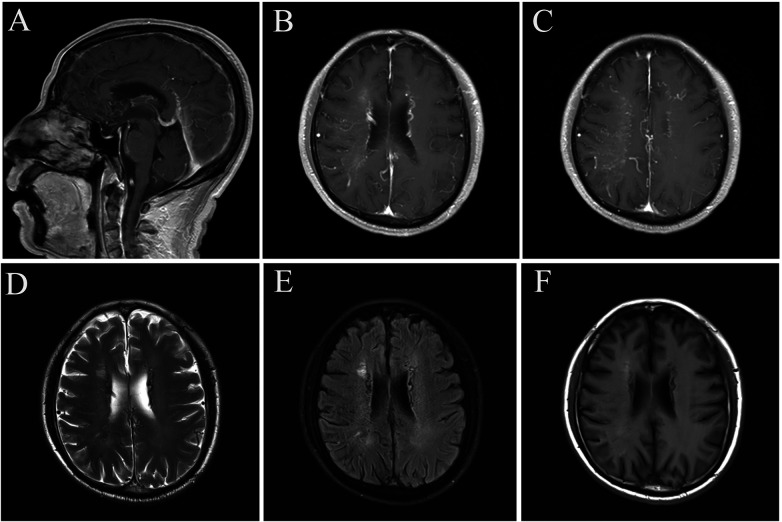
(A–F) cranial MRI imaging revealed multiple intricate and tortuous vascular signals within the brain, notably in the right occipital lobe and bilateral paraventricular regions. The signal within the superior sagittal sinus appeared non-uniform, and there was slight dilation of the major cerebral veins. Additionally, adjacent to the anterior horn of the right lateral ventricle, there was a patchy area displaying slightly shortened T1 and prolonged T2/FLAIR signals, with a mild enhancement effect.
